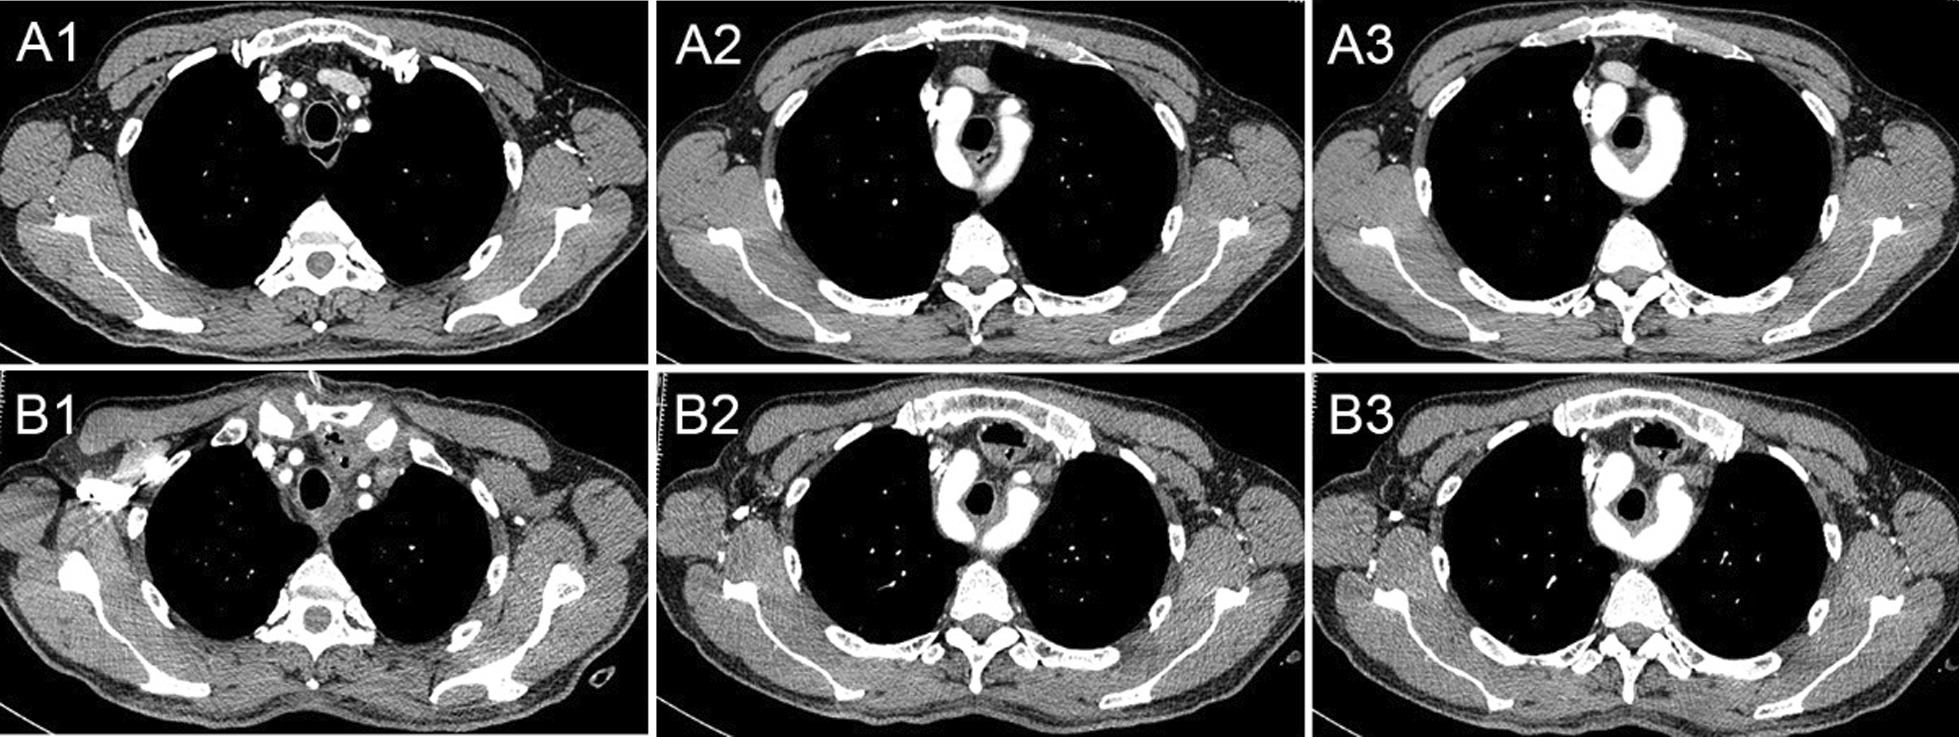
There was no brachiocephalic artery, and both the right common carotid artery and the right subclavian artery separately originated from the right aortic arch (RAA), and the left carotid arteries and subclavian arteries originated from the left aortic arch (LAA). The esophagus was displaced forward by the distal junction of both aortic arches and the descending aorta on the right side of the front of the thoracic vertebrae. The tumor was located in the esophagus between the aortic arch and the bifurcation of the trachea. CT Scans Show: Common carotid artery and subclavian artery on both sides (A1, preoperative;B1, postoperative); Vascular rings (A2, preoperative; B2, postoperative); Initial site of tumor (A3, preoperative; B3, postoperative)
